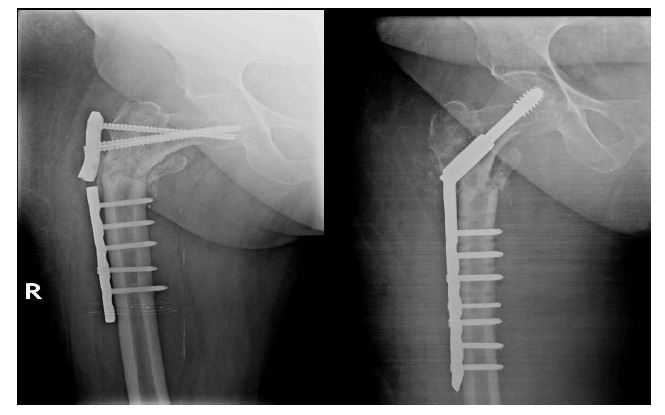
a) Post-operative radiograph of a 73 year old lady showing plate breakage due to varus collapse at 4months follow-up and fracture line crossing an empty hole ;b) Revision surgery with DHS.
